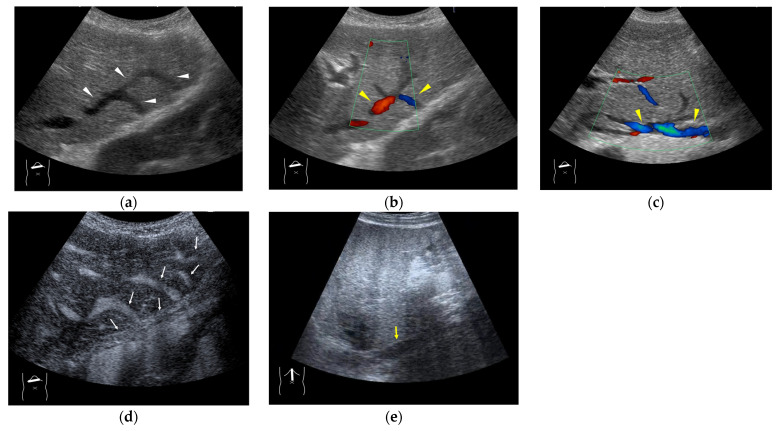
Representative case of Budd–Chiari syndrome (type 1): (a) B-mode US shows many venous collaterals (arrow heads) in the left lobe. (b,c) Color Doppler US shows slow flows in these collaterals (yellow arrow heads). (d) CEUS confirms communication between these collaterals and intracapsular collaterals (arrows). (e) It confirms no blood flow in obstructed IVC (yellow arrow).
